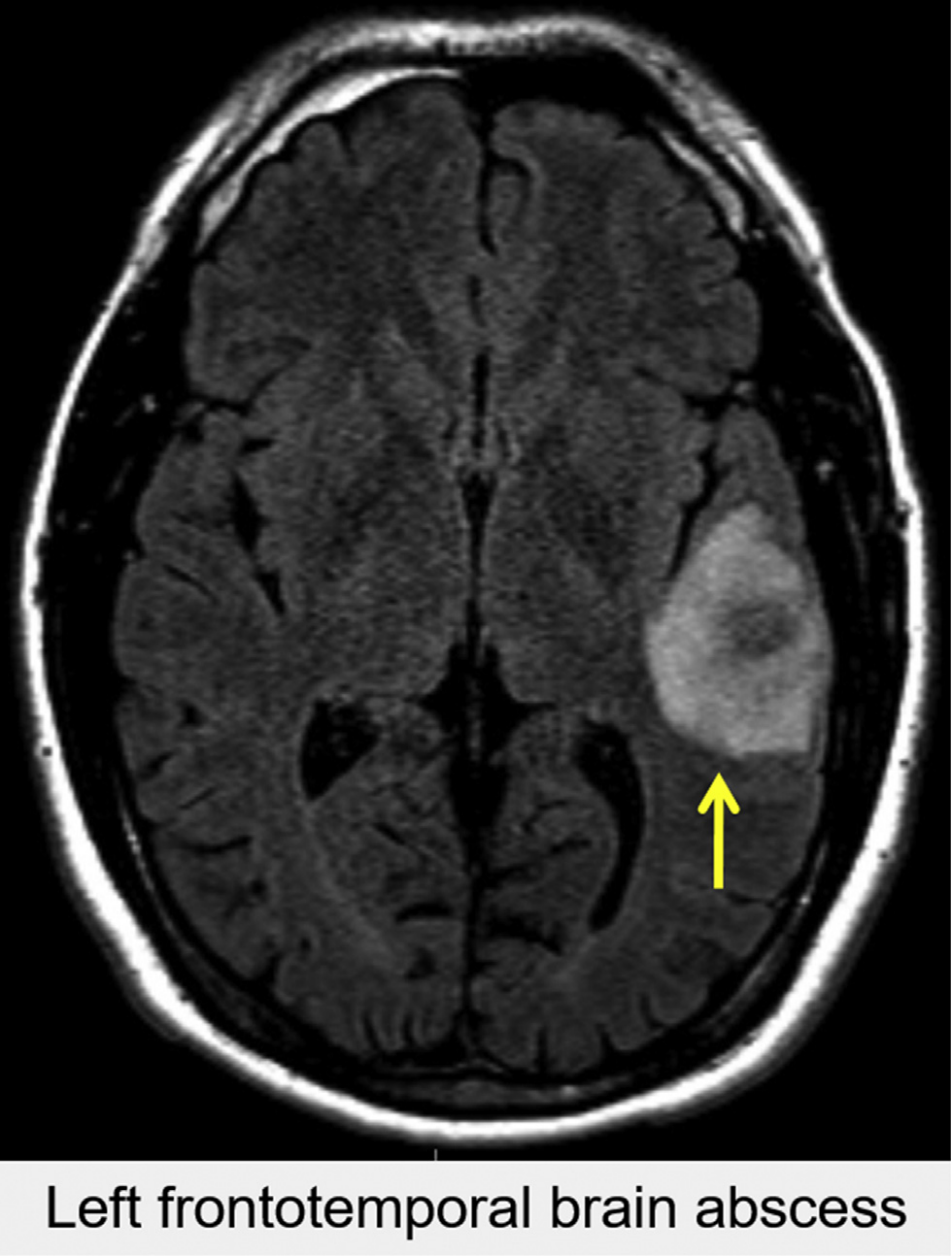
Case 5: R-SVC draining into the LA. Axial noncontrast head CT identified a round hyperdense lesion with a necrotic center located in the left temporal region of the patient's brain. This patient's clinical history and the appearance of the lesion is consistent with a brain abscess.
